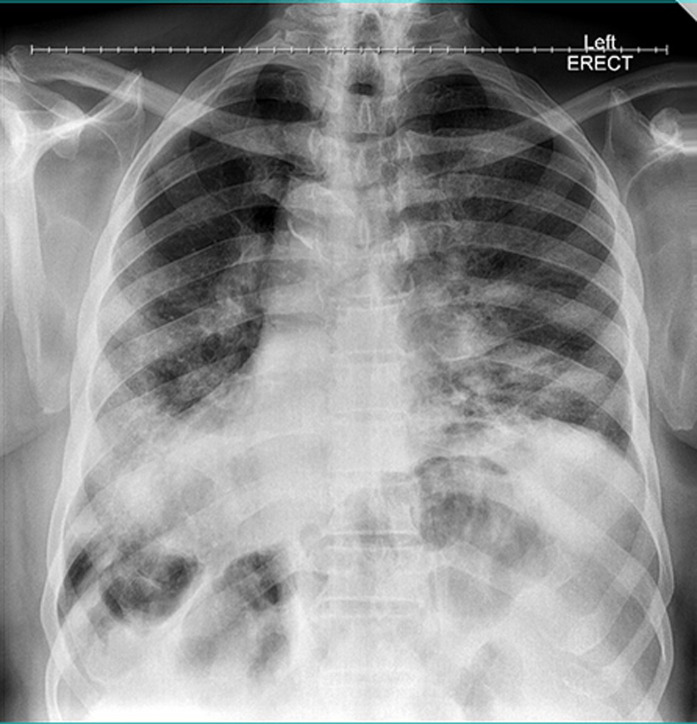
post-operative chest radiograph shows normal position of the left hemidiaphragm (red arrow) and normal position of the trachea and mediastinum (blue arrow) with right lung infiltrates
